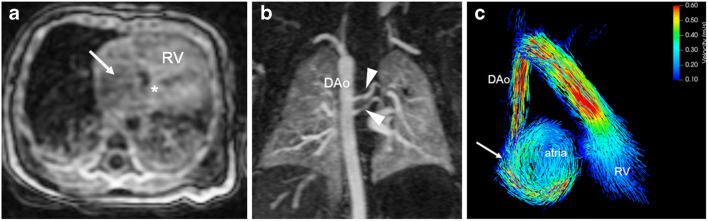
Heterotaxy syndrome associated with complete atrioventricular septal defect, insufficiency of the common atrioventricular valves, transposition of the great arteries, pulmonary atresia with non-confluent pulmonary arteries and multiple systemic collateral arteries originating from the descending aorta in a 1-day-old girl. Cardiac MRI was necessary for comprehensive anatomical overview preoperatively. a Axial 4-D flow MRI magnitude image demonstrates the quite impressive quality of 4-D flow raw magnitude images in neonates, resembling that of cine steady-state free precession sequences. It depicts the septal defect (*asterisk*), the right ventricular dilatation (*RV*) and a jet (*arrow*) in the right atrium caused by atrioventricular valve insufficiency. b Coronal MR angiography image shows two small aorto-pulmonary collateral arteries to the left lung (*arrowheads*) originating from the descending aorta (*DAo*). c Coronal 4-D flow pathlines reveal flow disturbances in the atria caused by insufficiency of the common atrioventricular valve resulting in a large vortex (*arrow*). The small aorto-pulmonary collaterals are not visualised
